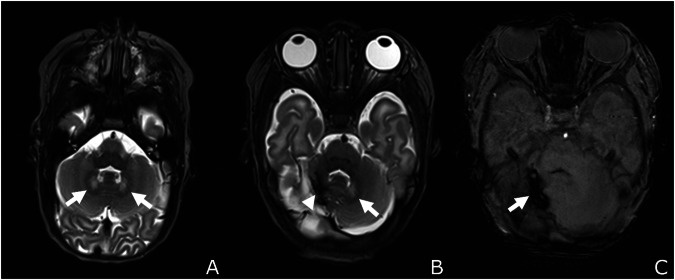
Cerebellar hemorrhage with involvement of the dentate nucleus (B, C). A Male preterm infant born at 27 weeks of gestation without cerebellar hemorrhage and normal appearance of the dentate nucleus on both sides (white arrows, T2-weighted sequence). B Female infant born at 28 weeks of gestation with a sizeable cerebellar hemorrhage and involvement of the right dentate nucleus (white arrowhead, left hemisphere: normal appearance of the dentate nucleus marked: white arrow, T2-weighted sequence) and C corresponding SWI-sequence (white arrow: cerebellar hemorrhage)
